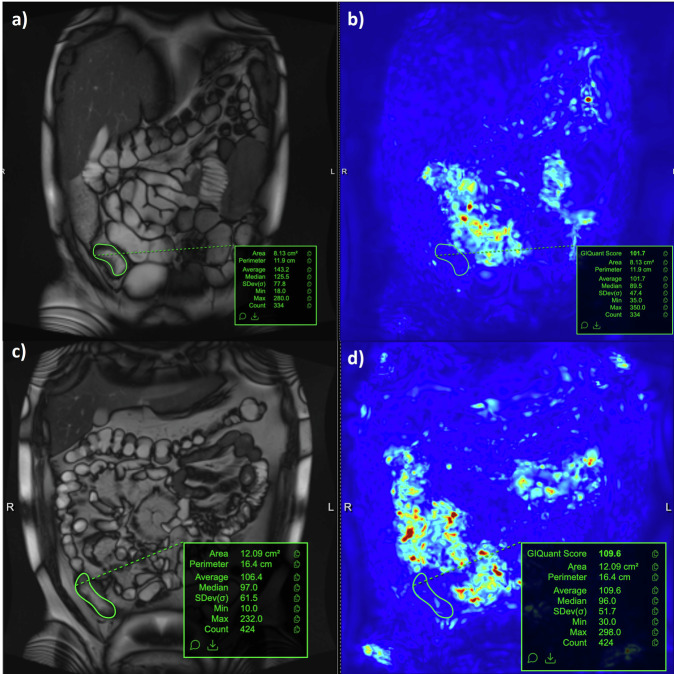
Technique for region of interest (ROI) placement for segmental small bowel motility MRI quantification. a, c Coronal T2-weighted images showing ROI placement by readers in the terminal ileum in 2 different cases. This ROI placement was selected to be as large as possible but also reproducible between studies at different time points within one patient. These ROIs were transferred to a calculated motility map (b, d) derived from the reference coronal T2-weighted images. This enables an average motility score to be derived (standard deviation of the Jacobean measured in artificial units)
